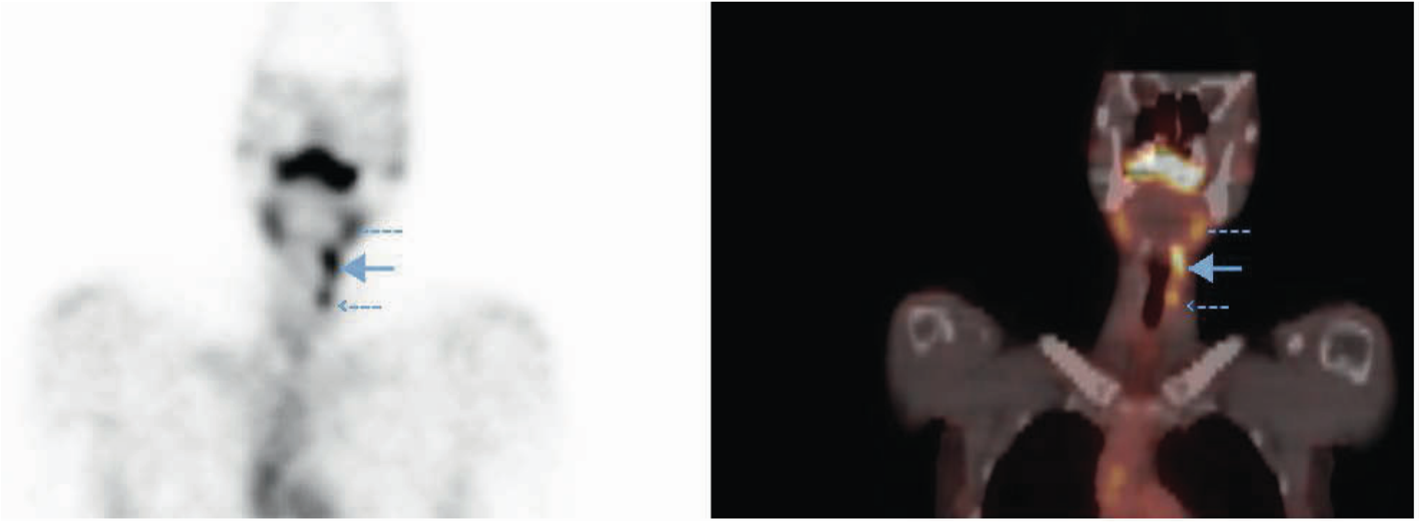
Corresponding SPECT and fused SPECT/CT images showing concerning cervical lesions. SPECT coronal slice (*left*) and fused SPECT/CT image (*right*) demonstrating abnormal foci of accumulation of ^99m^Tc0_4_
